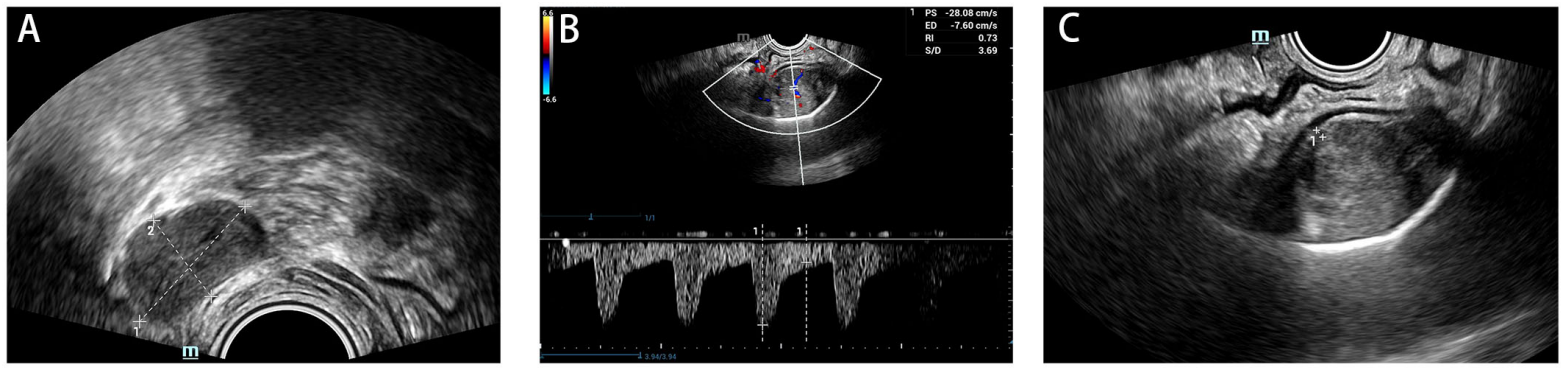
Two-dimensional ultrasonography at the time of diagnosis. A 51mm x 30mm x 71mm hypoechoic mass with heterogeneous internal echogenicity (A) striated blood flow information (B) was detected posteriorly in the rectum (C).
